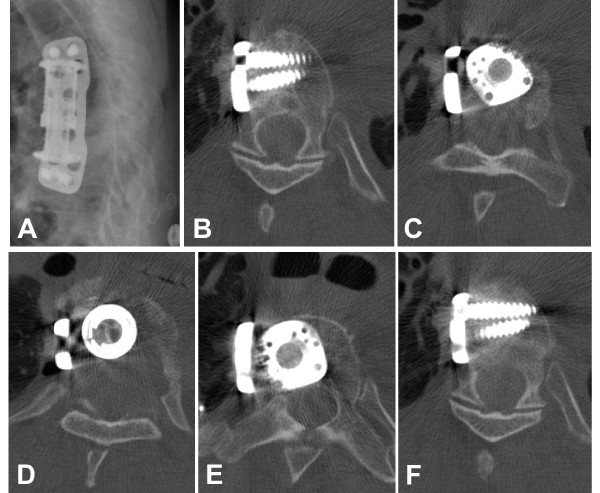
Status post anterior debridement through a right-side anterolateral thoracotomy, T7 and T8 corpectomy, anterior spinal canal decompression, and anterior fusion T6 through T9 by expandable cage, autologous bone graft, and anterolateral locking plate. Panel A shows the adequate restoration of the sagittal profile of the thoracic spine, on plain X-rays. The axial CT views demonstrate the adequate locking head screw placement of the anterolateral plate (panels B, F) and adequate expandable cage placement with spinal canal clearance (panels C, D, E).
